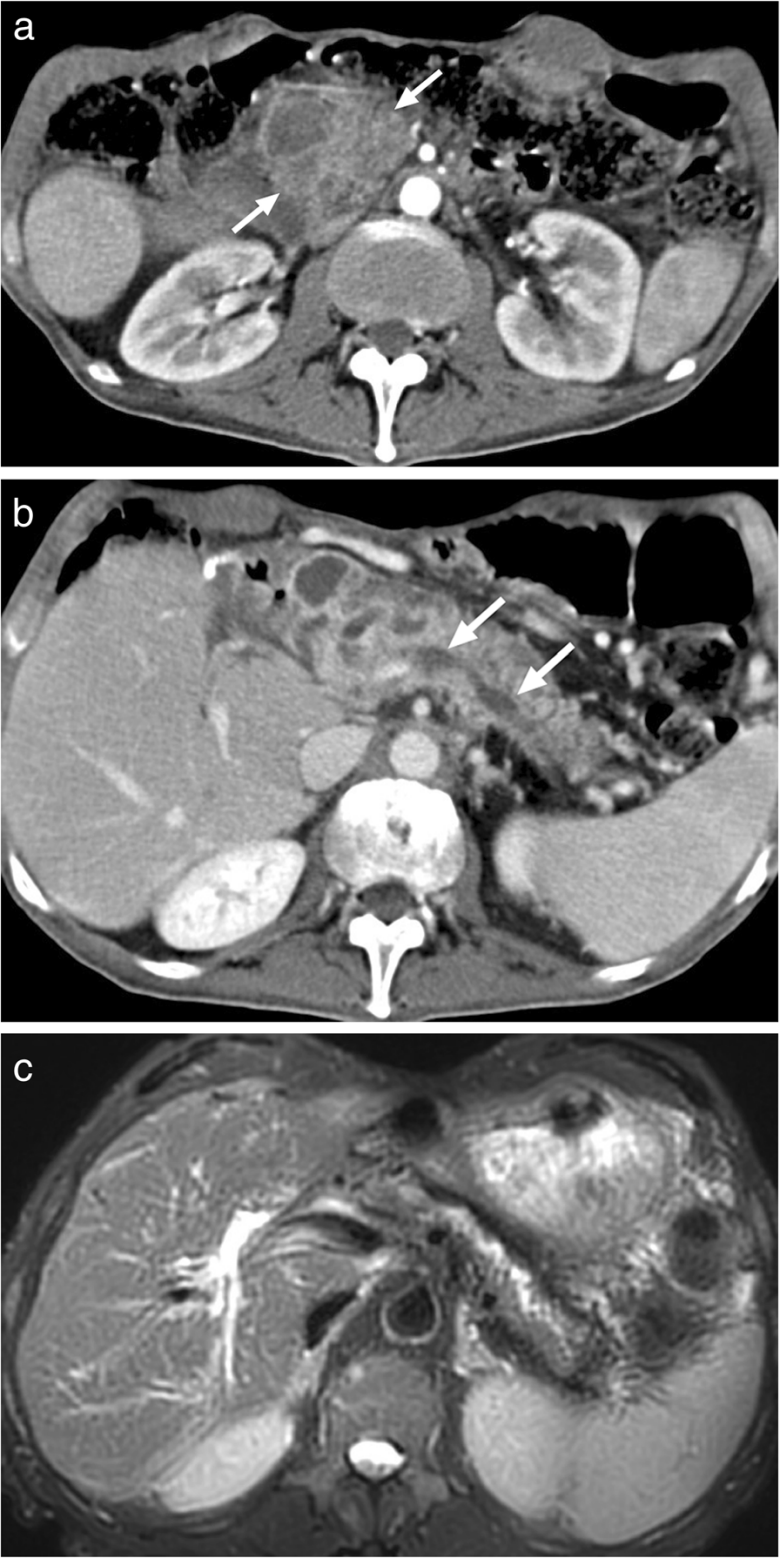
A 62-year-old male with no prior significant medical history presented with gradually increasing epigastric pain and weight loss. a Axial plane postcontrast late arterial phase CT image demonstrates diffusely thickened and heterogeneously enhancing pancreas (arrows) with ductal dilatation. b There was abrupt cessation of the pancreatic ductal dilatation (arrows) at the location of the pancreatic head mass. Image-guided percutaneous biopsy of the head lesion demonstrated TBC bacillus. The patient was then placed on multidrug antituberculosis treatment. Head, neck, and chest CT scans did not reveal any evidence of disease elsewhere. c Axial plane fat-suppressed T2W image 28 months after the initial scan showed significant regression of the pancreatic findings. At the time of this MRI scan, the patient was asymptomatic
